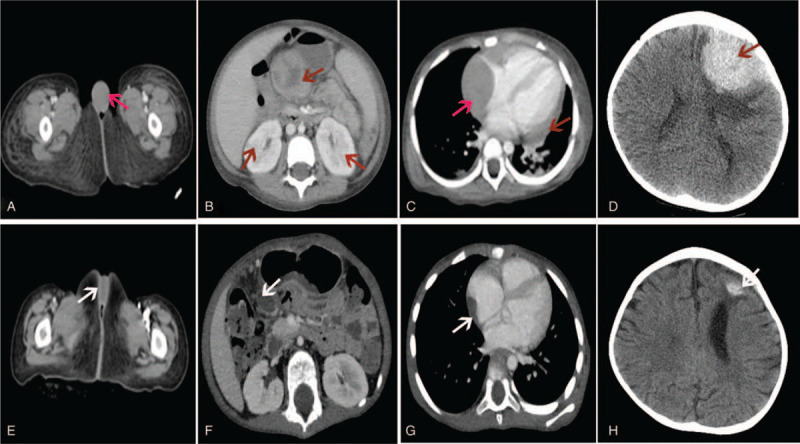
The findings in the clitoris, chest, abdomen, and brain before and after chemotherapy treatment. The location of the lesion was shown by the arrow. A. CT showed the mass in the clitoris. B. Abdomen CT showed an enlarged liver and spleen, multiple mass in the both kidneys. The enhancement CT scan of abdominal indicated a cystic mass on the neck of the pancreas which protruded outside the outline of the pancreas (red arrows). C. A chest CT showed a round soft tissue shadow (3.8 × 3.3 cm, red arrows) around the right atrium, with no obvious enhancement. The lesion compression was near the right atrium, and there is with few of pericardial effusion. D. The head CT showed a circular high-density shadow on the left forehead, with a size of about 5.3 × 4.2 cm and a circular low-density shadow around it. The lesion is showing mild enhancement. The lesions located in the clitoris (E), abdomen (F), chest (G), and head (H) were significantly reduced (white arrow). CT = computed tomography.
